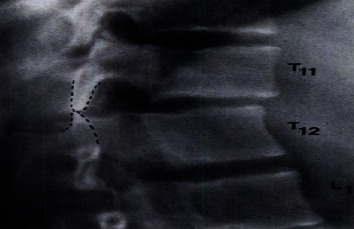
Distraction injury scanned by CT scan (showing damage occurrence at the 11^th^ and 12^th^ thoracic vertebrae).
